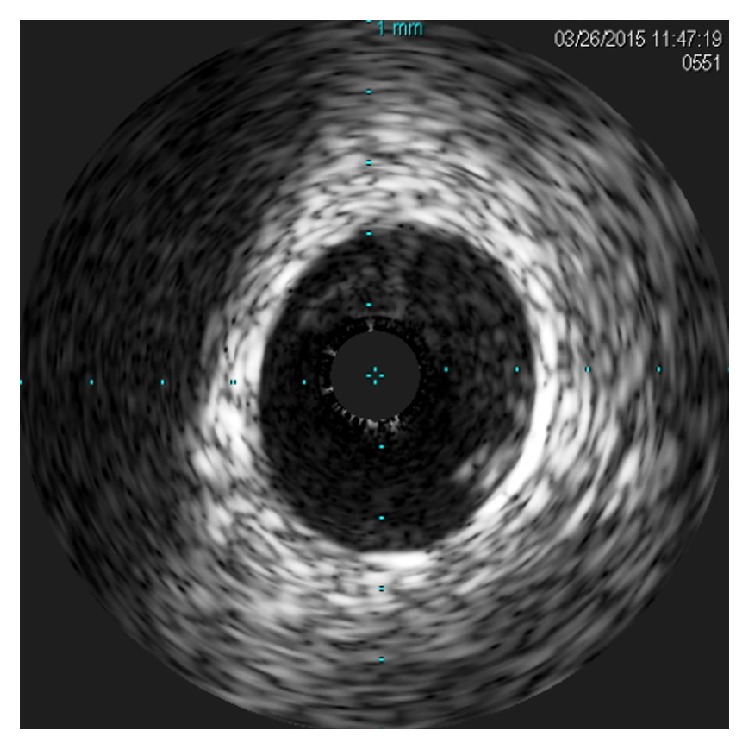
Intravascular ultrasound (IVUS) showing thrombus in proximal LAD, no evidence of dissection.
